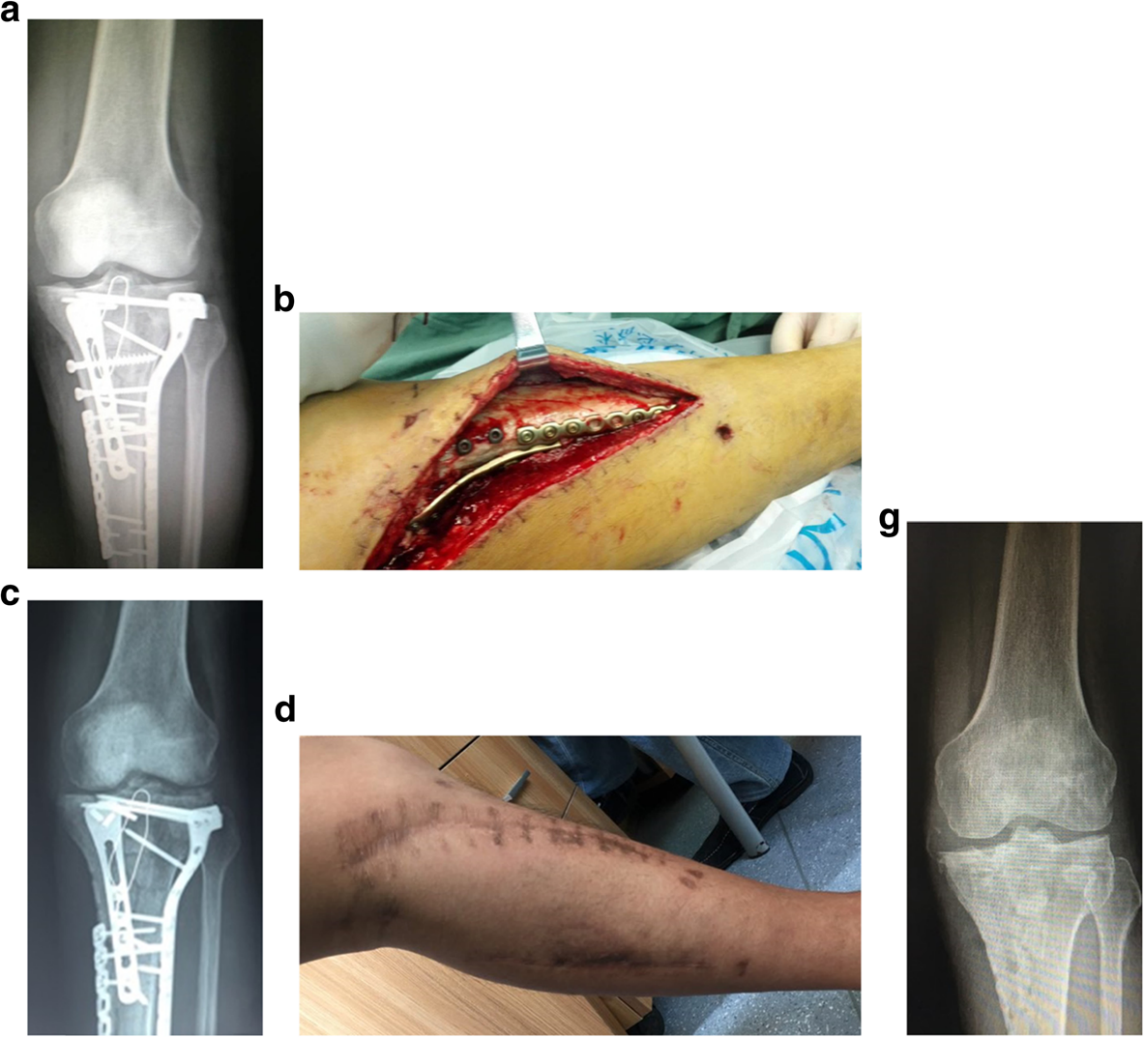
Stable hardware + bone not healed Cierny–Mader 2 type = retain hardware, debridement, soft-tissue coverage, bone healed, then hardware removal. a Radiographs of primary internal fixation. b Debridement. c Radiographs 1 month after treatment. d–f After 12 months of treatment, the wound was stable. g Radiograph 12 months after treatment
